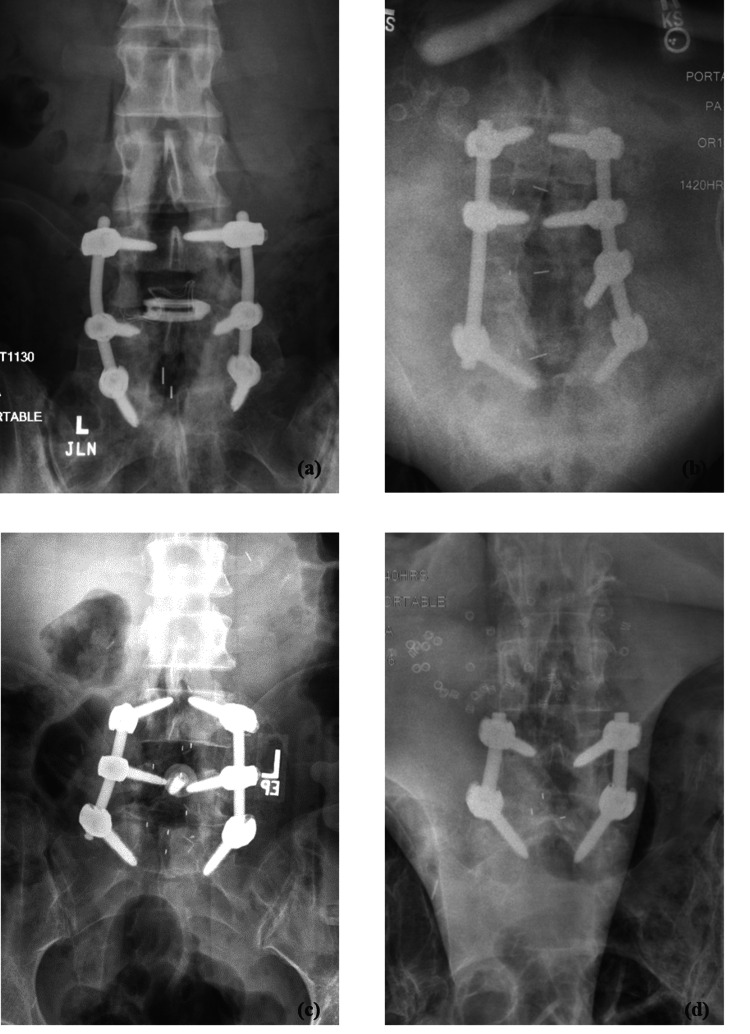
Anteroposterior (AP) radiographs - examples of (a) true positive (subject 65), (b) false negative (subject 7), (c) false positive (subject 113), (d) true negative (subject 3)
